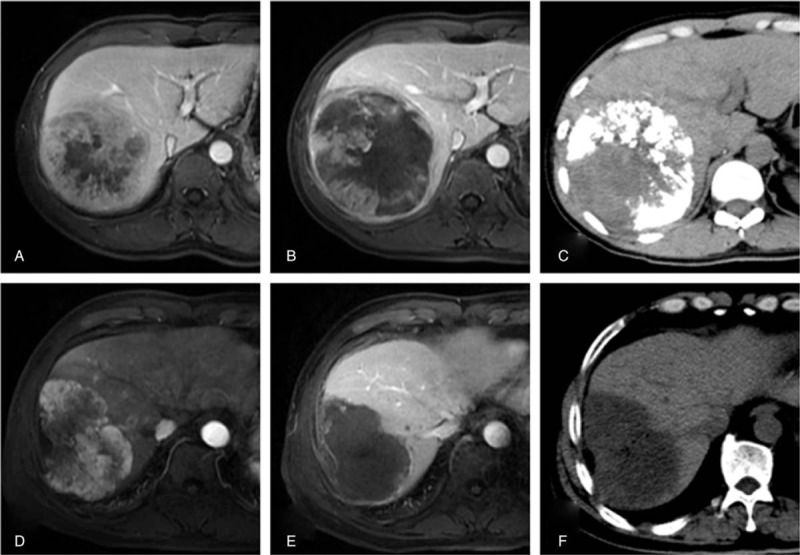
Results of abdominal computed tomography (CT) and magnetic resonance imaging (MRI) and the changes in tumors in a representative case before and after transarterial chemoembolization (TACE). MRI with tumor enhancement pre-TACE in a patient later treated with GSP (A) and Lipiodol (D). Note the enhanced integrity of the tumor in both A and D. MRI with tumor enhancement and CT scan in the patient treated with Lipiodol (B and C) and gelatin sponge particle (E and F). Partial enhancement can be seen in Figure B. There is no enhancement in the tumor in Figure E, the distribution of Lipiodol in the tumor is shown in Figure C. A low-density shadow is found in the tumor (F).
